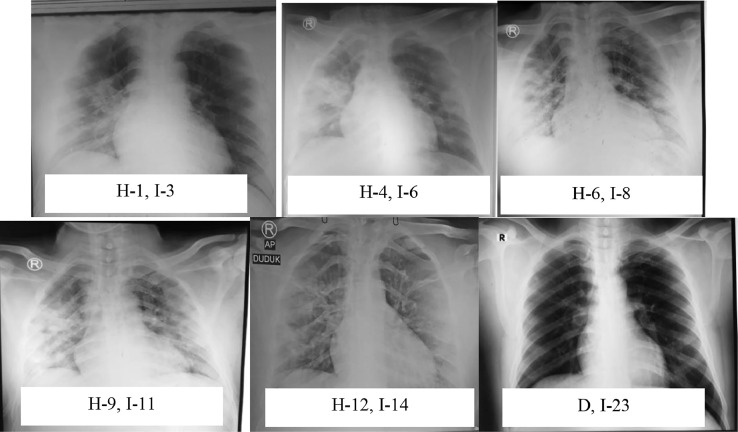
Serial of chest X-ray and chest CT scan. H = day of hospitalization, I = day of illness, D = discharged from isolation room.
